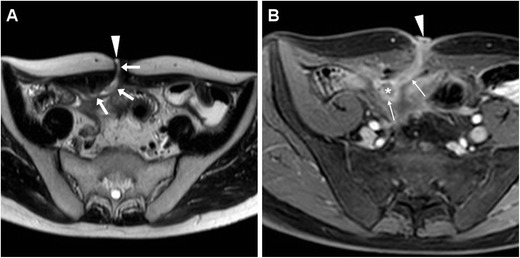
Spontaneous ECF in a 37-year-old man with stricturing and fistulising CD, shown at MR-enterography as a fluid-like T2-hyperintense track (arrows in A) coursing from an ileal loop through the abdominal wall, and finally reaching the EFO (arrowheads). Post-gadolinium fat-suppressed T1-weighted acquisition (B) showed mucosal hyperenhancement along ECF track (thin arrows) and at site of internal opening (*). Surgical treatment required fistula debridement plus stricturoplasty
